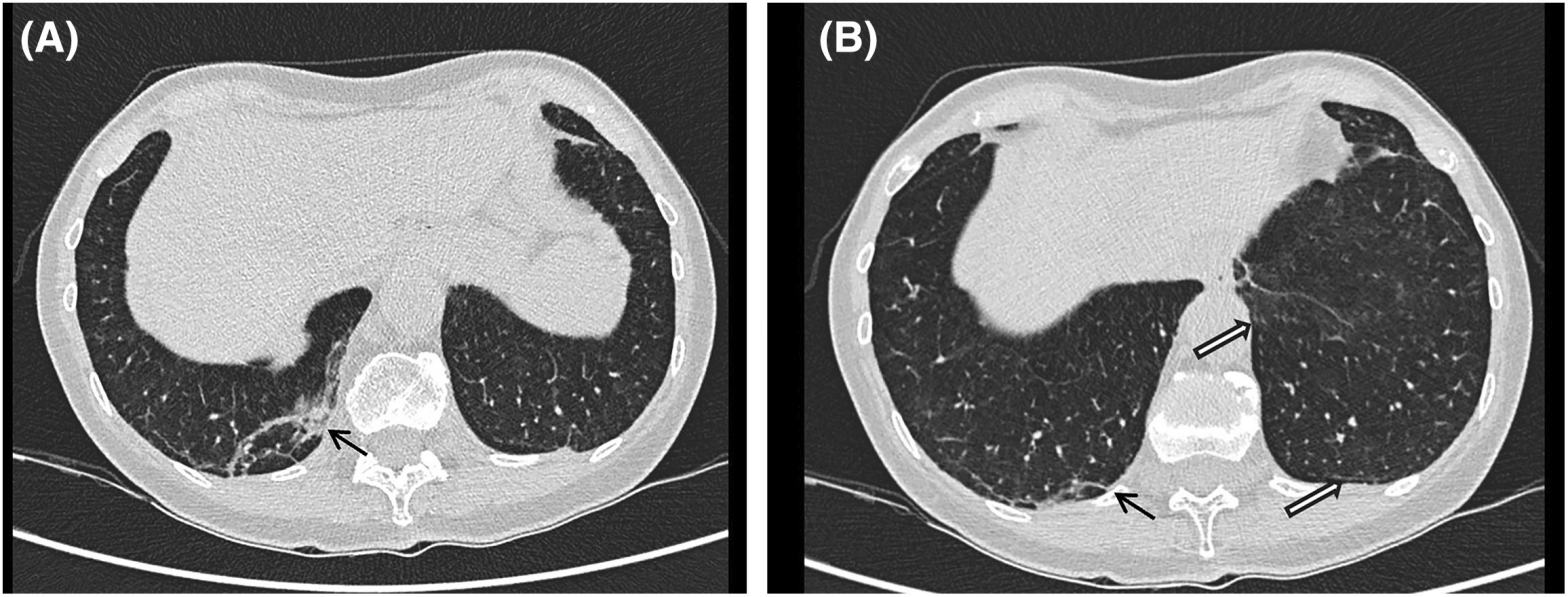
(a) Lung HRCT 2019: Tiny alterations, interpreted as mild ILD, are appreciable mainly at lung periphery of middle and lower zone (white arrows). (b) An atelectatic band is also present in the right lower lobe (black arrows).
